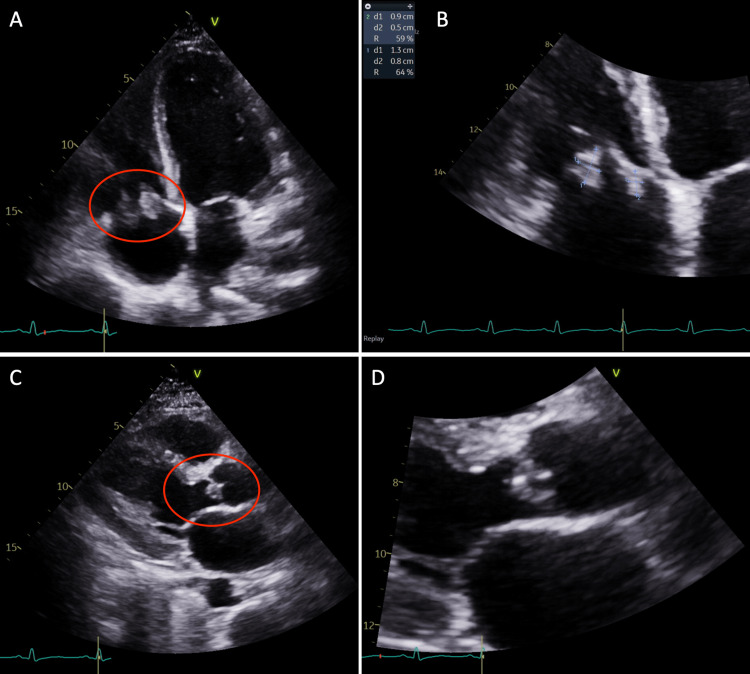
Transthoracic echocardiogram apical four-chamber view (A) and magnified view of the tricuspid valve (B) revealing echodensities measuring 0.8 cm × 1.3 cm and 0.9 cm × 0.5 cm on the atrial side of the anterior and septal tricuspid valve leaflets. The parasternal long-axis view (C) and magnified view of the aortic valve (D) revealing a <1 cm echodensity on the ventricular side of the noncoronary cusp of the aortic valve.
